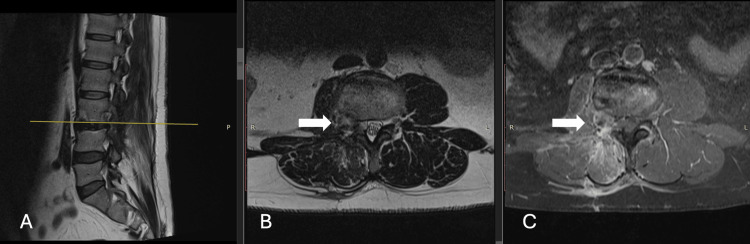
Sagittal (A), axial (B) and axial T1 Gd (C) MRI views Images after the second surgery show the L3-L4 intersomatic cage (lateral lumbar interbody fusion (LLIF)) and preserved facet joint with the tumor. No paraspinal tumor is observed. Gd: gadolinium
